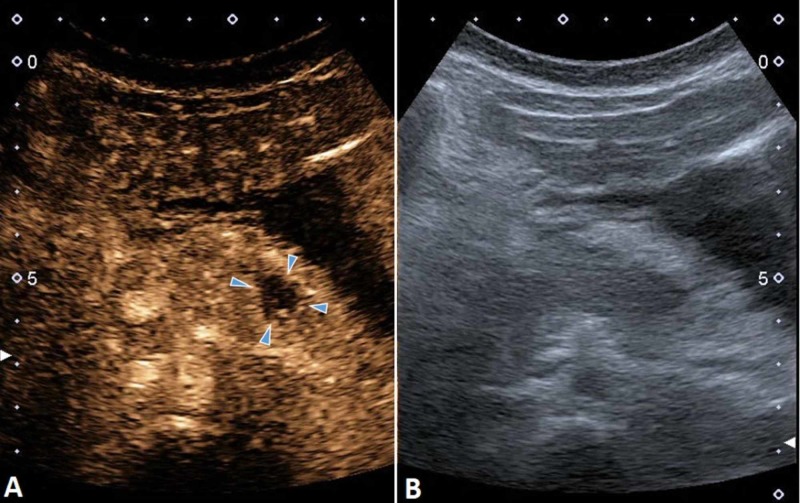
CEUS (A) demonstrating a 14 mm perfusion defect in the tail of the pancreas (arrowheads), which was not clearly seen on the B-mode US (B). CEUS, contrast-enhanced ultrasound US, ultrasound
